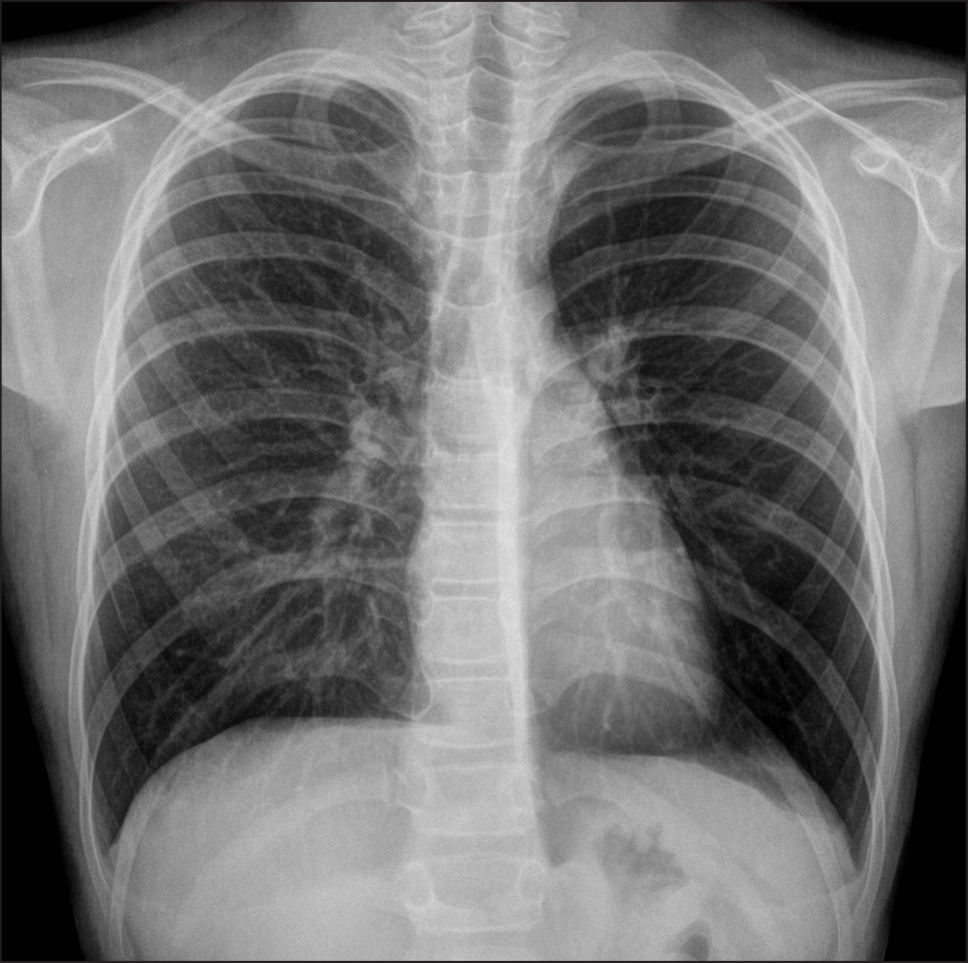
Standard chest radiograph.
